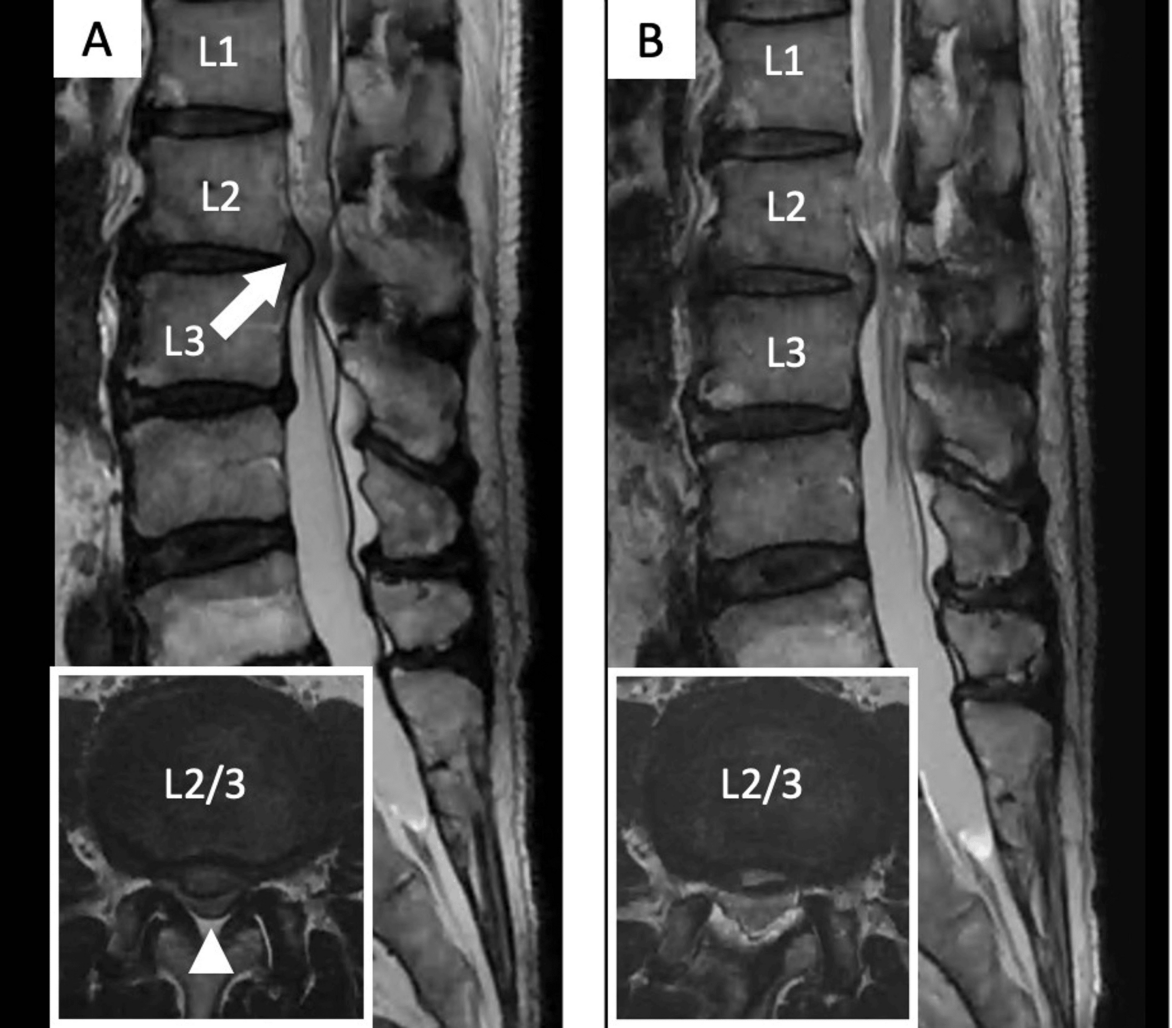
Case 1: Preoperative and postoperative magnetic resonance imaging (MRI). (A) Preoperative MRI reveals a central-type disc herniation (arrow) with spinal canal stenosis (arrowhead) compressing the dural sac at L2–L3. (B) Postoperative MRI shows complete laminectomy and adequate removal of the herniated disc.
